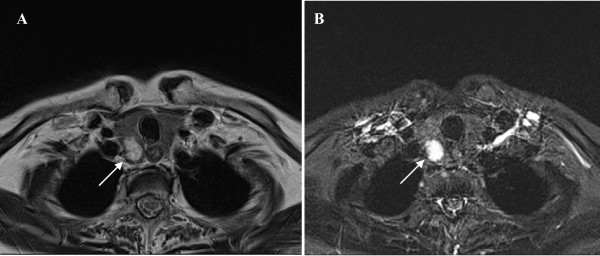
MRI scan of the neck. (A) T2 blade sequence. (B) T2 blade sequence with contrast enhancement. The thyroid nodule of the right lobe is indicated by a white arrow. (Images were provided by the Institute of Radiology, University of Regensburg).
